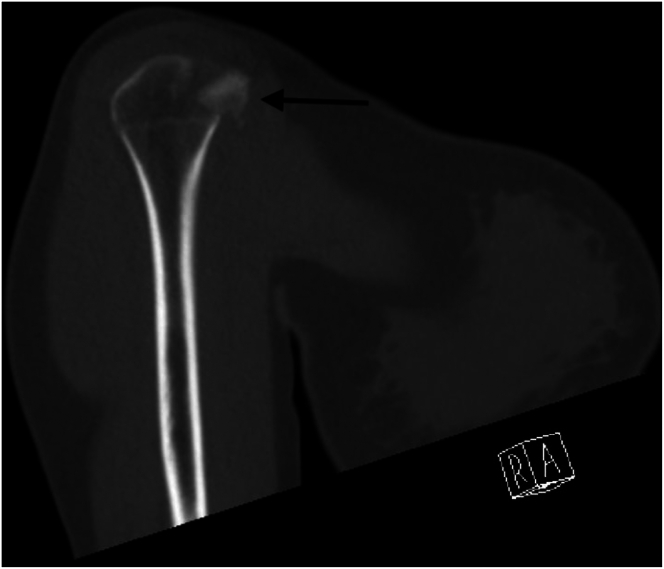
Coronal computed tomography image revealing fracture morphology and displacement of the lesser tuberosity fragment (arrow). (R, right shoulder).
